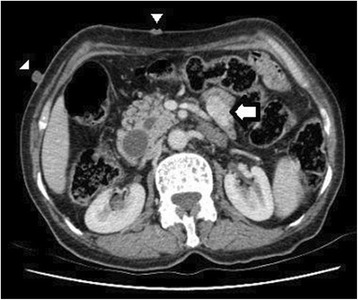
Contrast enhanced computed tomography of abdomen showing the duodenal gastrointestinal stromal tumor (arrow) and neurofibroma (arrowhead)
